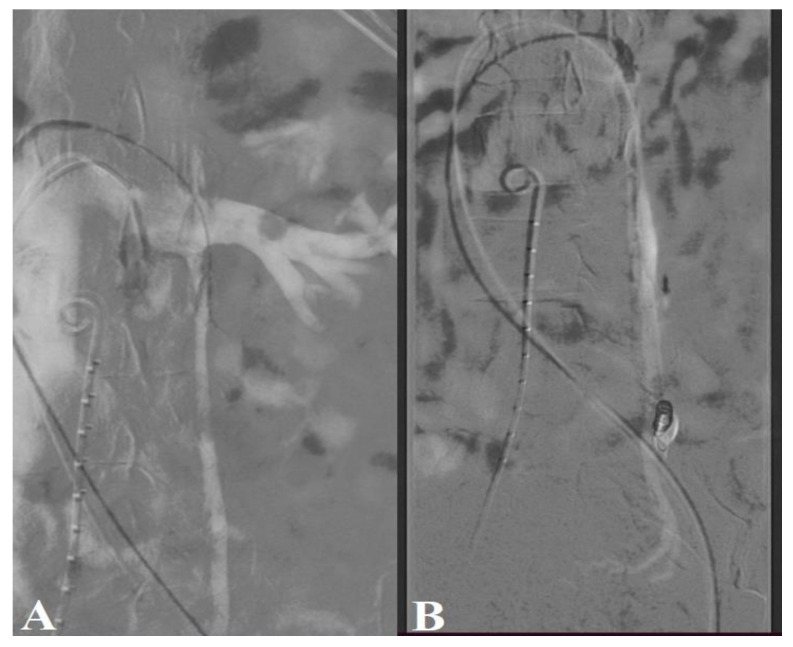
CT-guided endovascular embolization. The left ovarian vein was catheterized via transfemoral approach, respectively, through the left renal vein (A). In venograms performed during the Valsalva maneuver, left ovarian vein insufficiency with paraovarian varices was detected (B).
